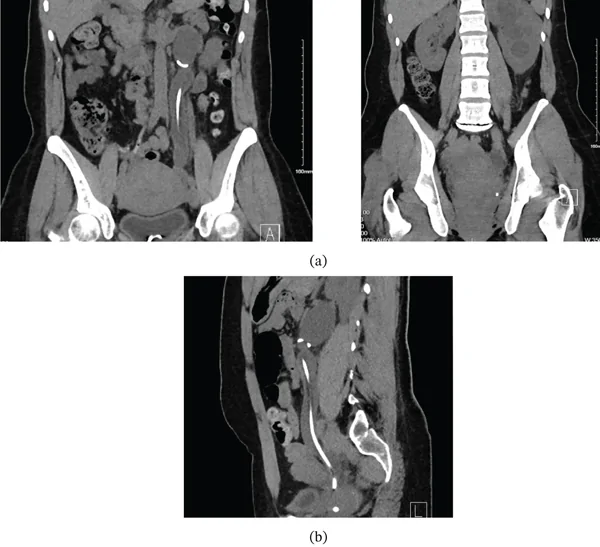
CT imaging after ureteral stent placement. (a) Coronal images after stent placement. (b) Sagittal images after stent placement.
